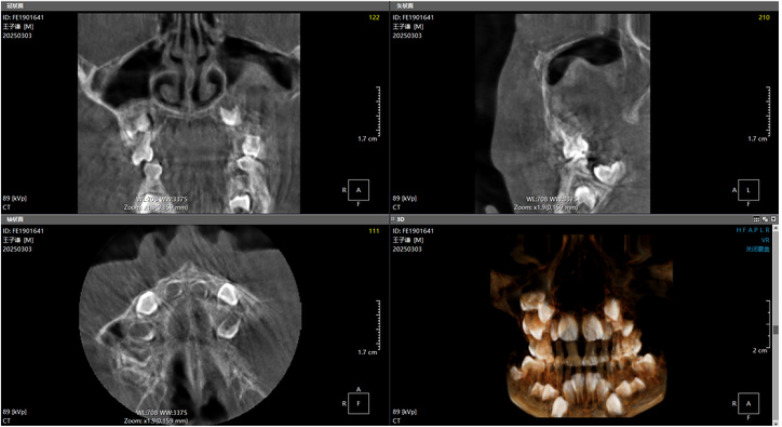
Postoperative CBCT image (1).
